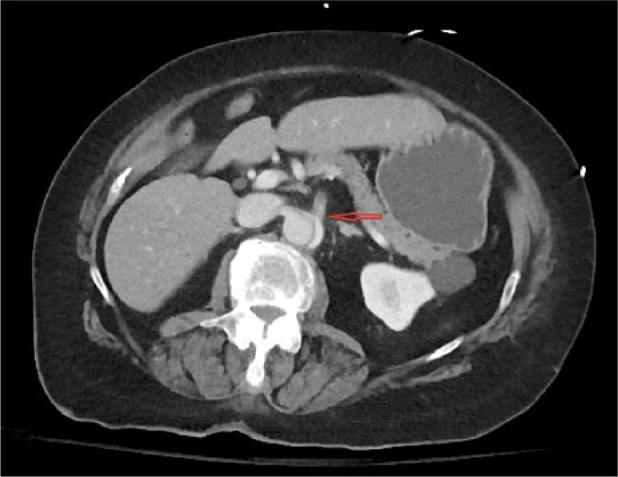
Arrow indicating to dissection flap extending to superior mesenteric artery (SMA) on contrast enhanced CT.
